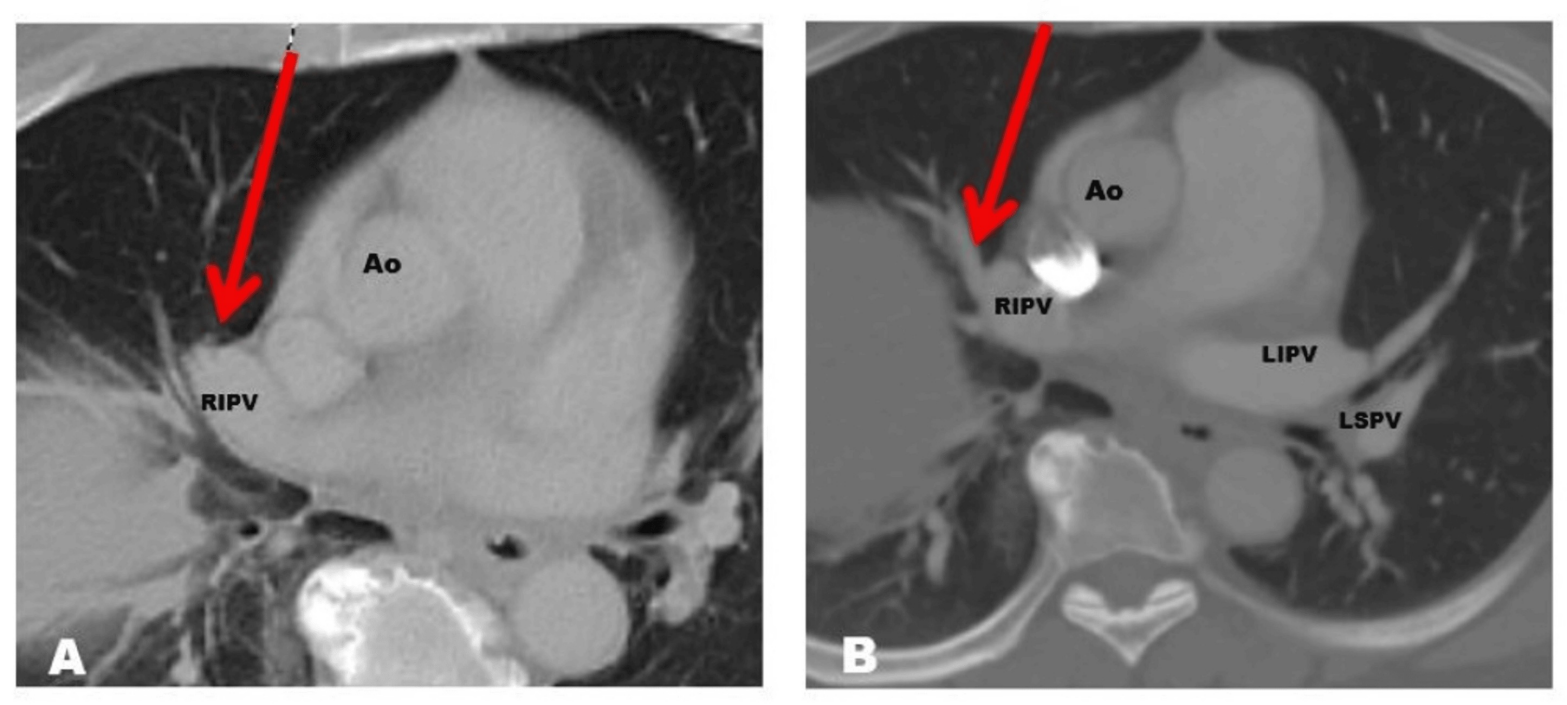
Chest CTA On the left (4A), dedicated chest CTA performed at baseline with occlusion of the RIPV (red arrow). On the right (4B), follow-up chest CTA performed at 12 months with DOAC treatment shows resolution of the RIPV thrombus. RIPV: right inferior pulmonary vein; CTA: computer angiography; DOAC: direct oral anticoagulant
